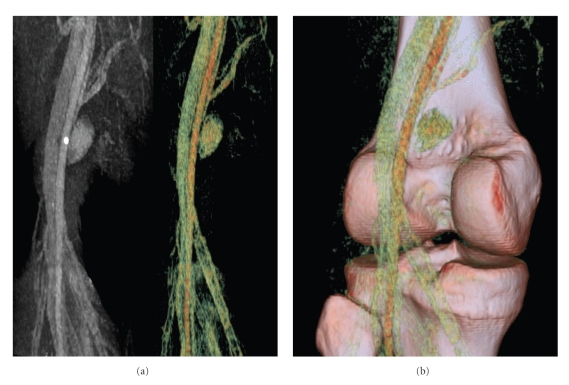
Angio-Computed Tomography* (ACT) scan reconstruction using maximal intensity projection (MIP), volume rendering (VR) technique, and 3D showing the popliteal venous aneurysm in the left popliteal fossa.
